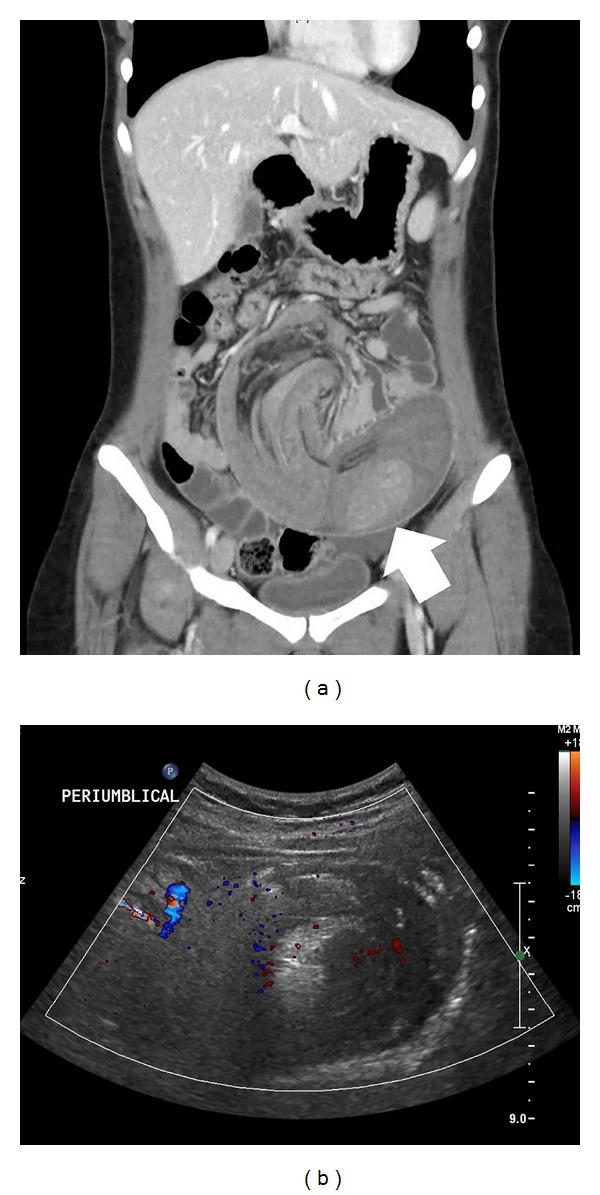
Abdominal pelvic computed tomography and ultrasonography. (a) Coronal view of the CT scan shows a long segment of jejunojejunal intussusception and polyps within the jejunum (white arrow). (b) Sonographic finding of a target sign suspected to be intussusception.
